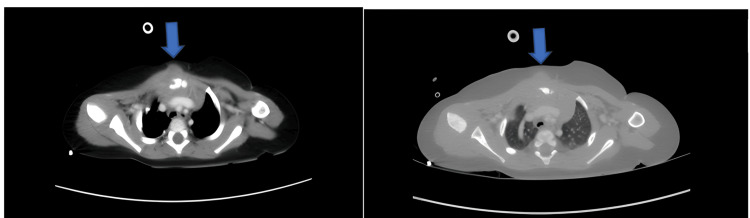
Thoracic CT image of the patient taken in our hospital
